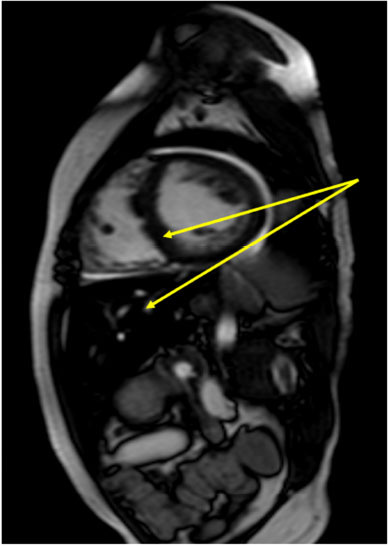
T2* Mid ventricular short axis acquisition in cardiac MRI showing that both the heart (Interventricular septum) and the liver have reduced and equal signal intensity (arrows), suggestive of severe iron overload.
